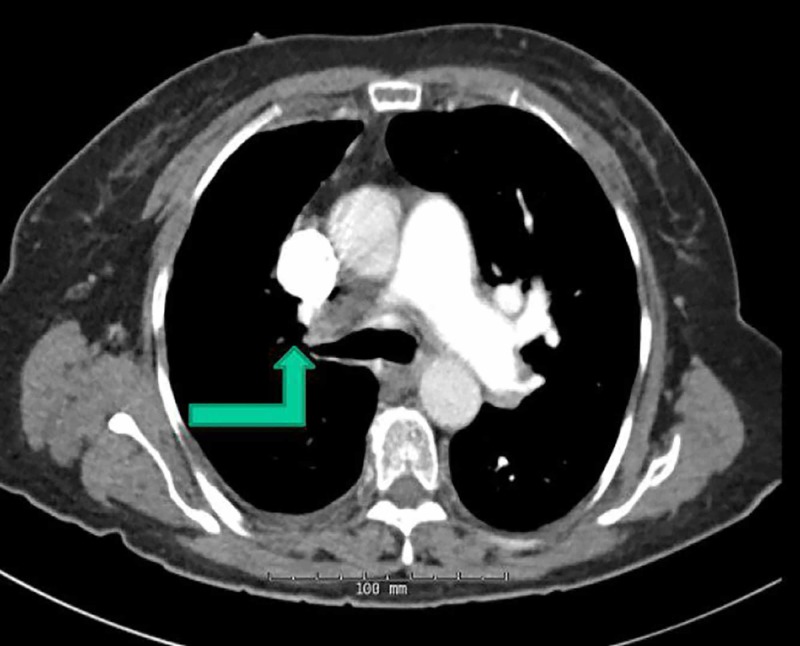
CT angiography showing significant atresia of right pulmonary artery (green arrow)
